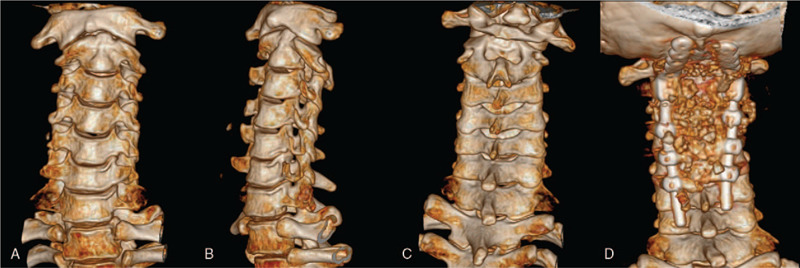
Three-dimensional CT reconstruction. (A–C) Preoperative 3-dimensional CT showed a slight anterior lateral dislocation of the atlas and an unstable atlantoaxial position. (D) Postoperative 3-dimensional CT. CT = computer tomography.
